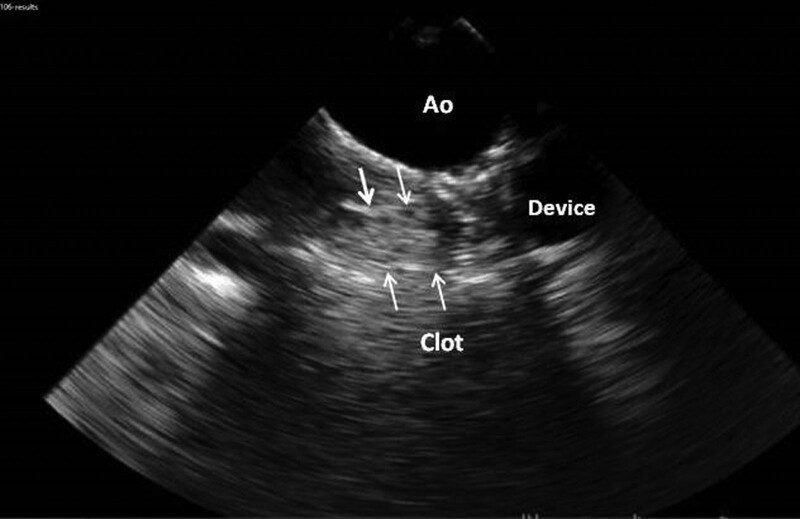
Transesophageal echocardiography images. It shows the clot formation back to the device in the vertical vein.
